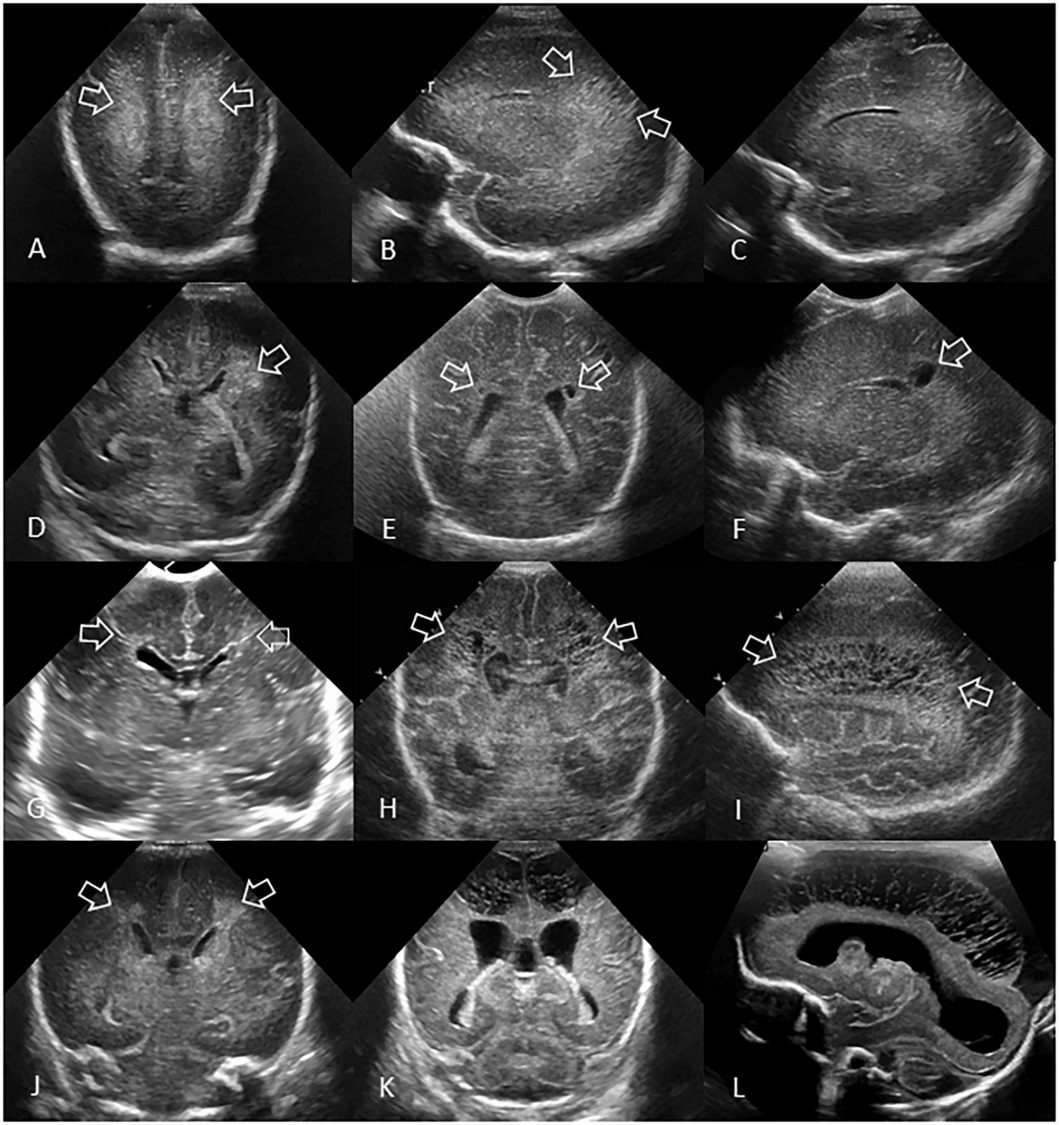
Spectrum of ischemic white matter injury. (A–C) Grade 1 WMI. Ultrasound scan in a preterm infant (GA 26 weeks) with transient abnormal white matter echodensities. (A,B) Coronal and left parasagittal scans show inhomogeneous moderately increased echogenicity (“flaring” or PVE) of the parietal periventricular white matter (arrows). No IVH. (C) Parasagittal scan 5 weeks later shows full resolution of the echodensities. White matter echogenicity is now homogeneous and normal, and no cysts developed. (D–F) Grade 2 WMI. Ultrasound scan in a preterm infant (GA 27 weeks). (D) Coronal scan at the level of the body of the lateral ventricles shows asymmetric inhomogeneous PVE (“flaring”) in the left parietal periventricular white matter (arrow). (E,F) Coronal and left parasagittal scans 9 weeks later showing small localized cystic lesions in the fronto-parietal white matter of both hemispheres (arrows). The tiny, right-sided cysts developed despite any convincing increased white matter echogenicity detected on early cUS exams. (G–I) Grade 3 WMI. Ultrasound scan in a preterm infant (GA 30 weeks with giant omphalocele partially repaired at day 4 of birth) with significant WMI. (G) Coronal scan 3 days after birth, at the level of the frontal horns of the lateral ventricles, shows only limited patchy areas of echodensity (arrows) not with certainty distinguishable from physiologic frontal echogenic areas in the periventricular white matter. No IVH. (H,I) Coronal and left parasagittal scans 4 weeks later show evolution of the WMI with extensive cystic lesions extending from the frontal white matter to the posterior parietal white matter (arrows) not expected by the only limited patchy echogenicity seen on day 3. The subcortical white matter is not involved. Dilated ventricles are passive in origin and resulting from tissue volume loss. (J–L) Grade 4 WMI. Ultrasound scan in a preterm infant (GA 25 weeks) with significant WMI. (J) Coronal scan 5 days after birth, at the level of the body of the lateral ventricles, shows patchy areas of echodensity (arrows) in the periventricular frontoparietal white matter. No IVH. (K,L) Coronal and right parasagittal scans 3 weeks later show evolution of the WMI with extensive cystic lesions extending from the frontal to the posterior parietal white matter. The progression of WMI could be recognized thanks to serial cUS exams as its severity was not expected by the limited patchy echodensity originally seen on day 5. Dilated lateral ventricles are passive in origin and result from tissue volume loss.
